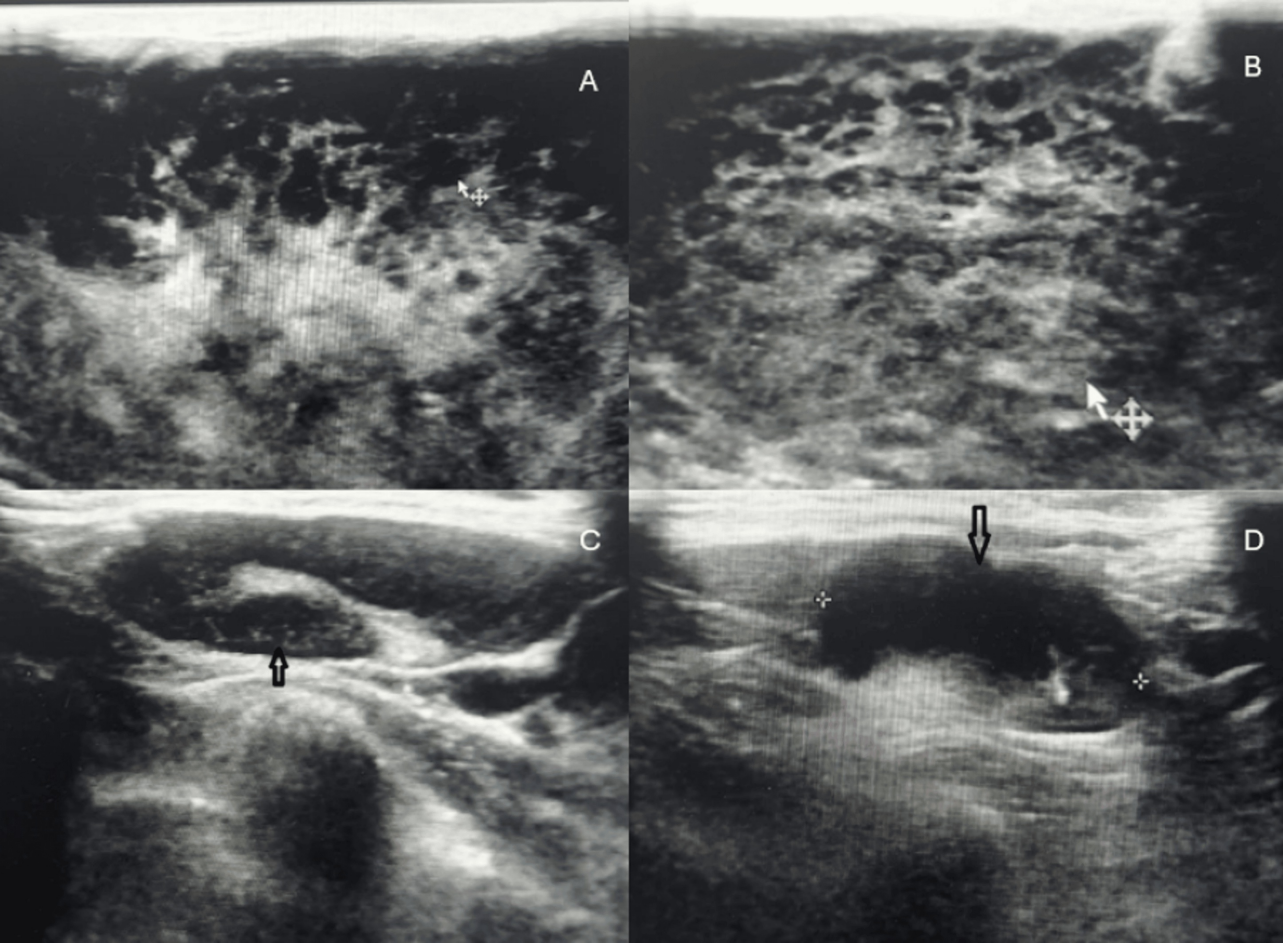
Breast US showing bilateral heterogenous masses (A, B) and enlarged axillary LN (C, D). US: ultrasound; LN: lymph nodes.
